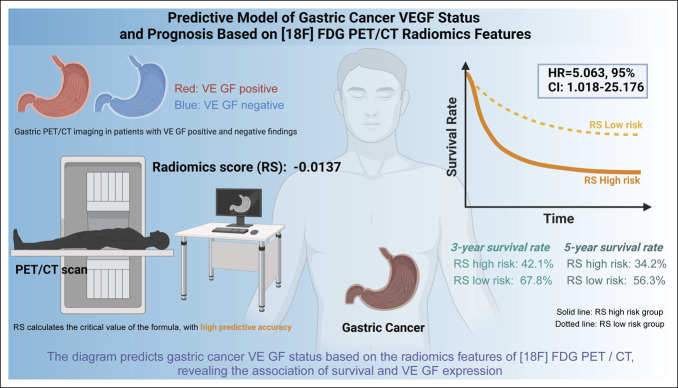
Predictive model of gastric cancer VEGF status and prognosis based on [18F] FDG PET/CT radiomics features. CT, computed tomography; FDG, fluorodeoxyglucose; HR, hazard ratio; PET, positron emission tomography; VEGF, vascular endothelial growth factor
